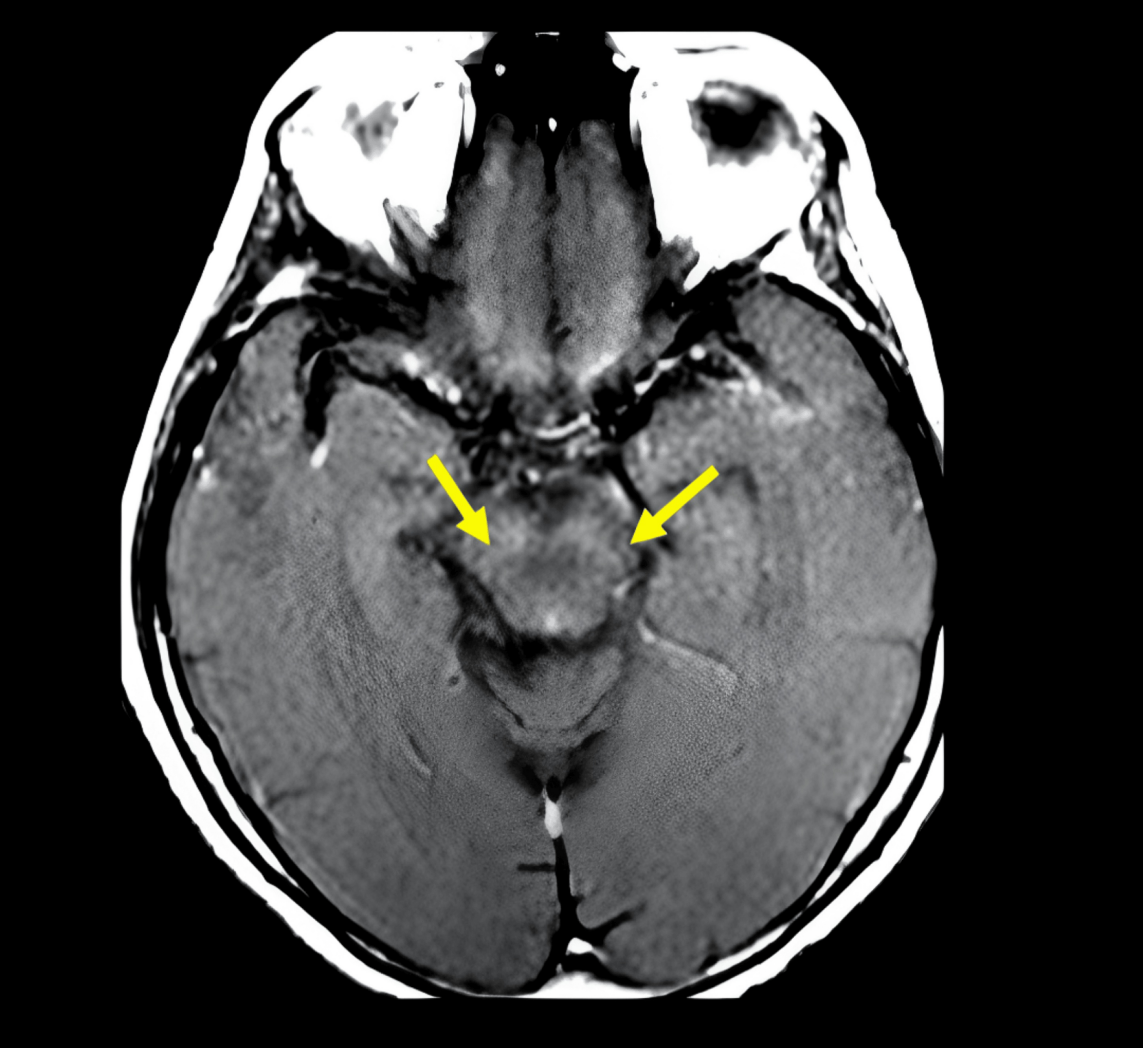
MRI showing a mesencephalic lesion and thinning of the substantia nigra
